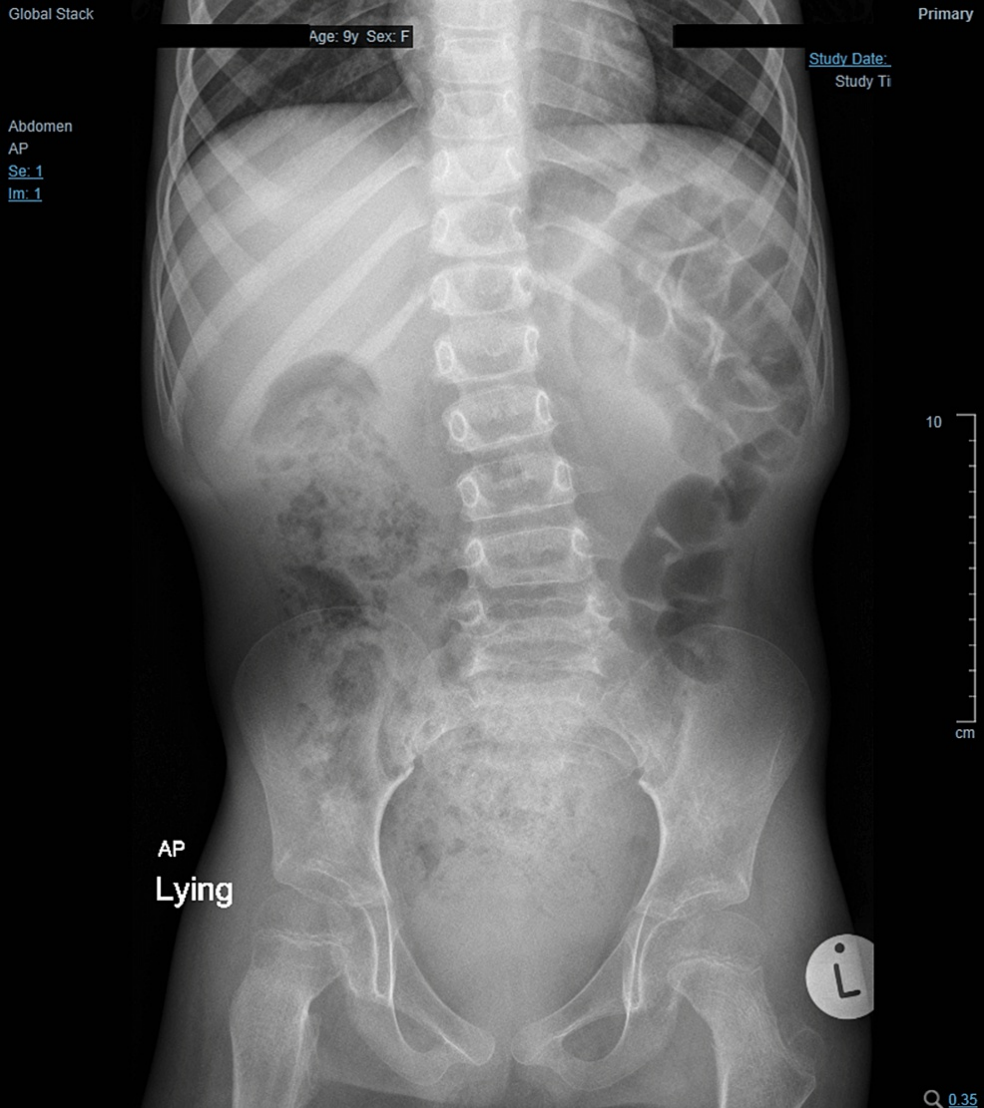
Post-enema radiograph showing the resolution of the fecaloma and dilated bowel loops
